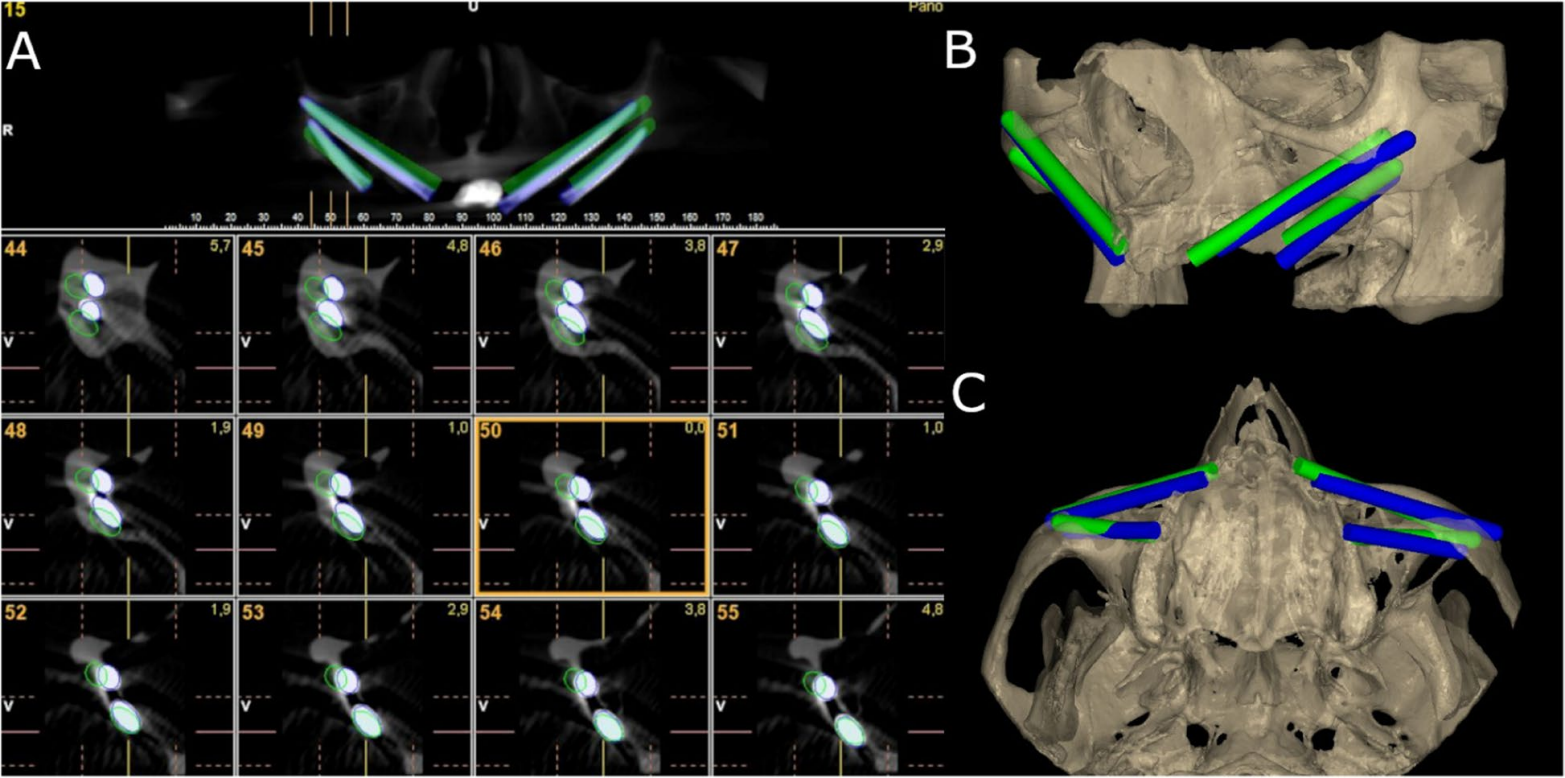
A CBCT images and B, C 3D render of the measurement procedure comparing the preoperatively planned (green cylinders) and postoperative implants (blue cylinders) placed on the experimental models
